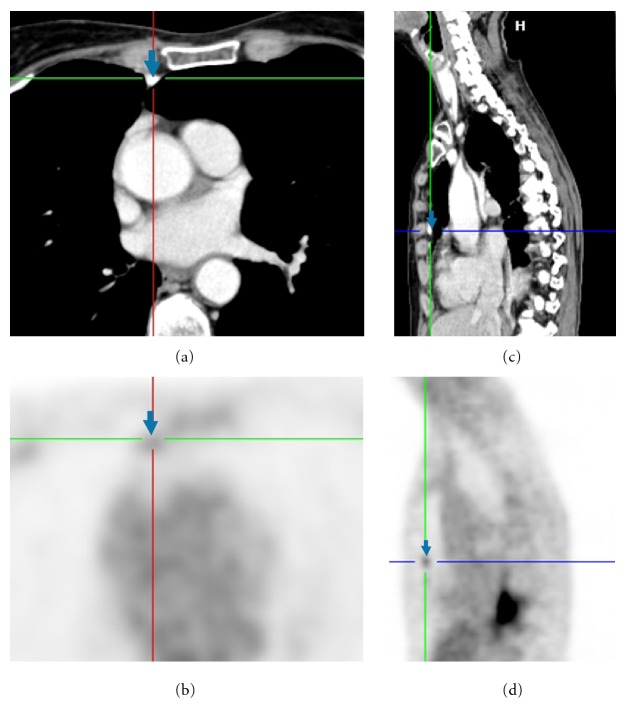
A Patient with avid and high-attenuation right internal mammary lymph node (arrow) on axial CT (a), axial PET (b), sagittal CT (c), and sagittal PET (d) images. Chest CT images prior to talc pleurodesis are not available.
